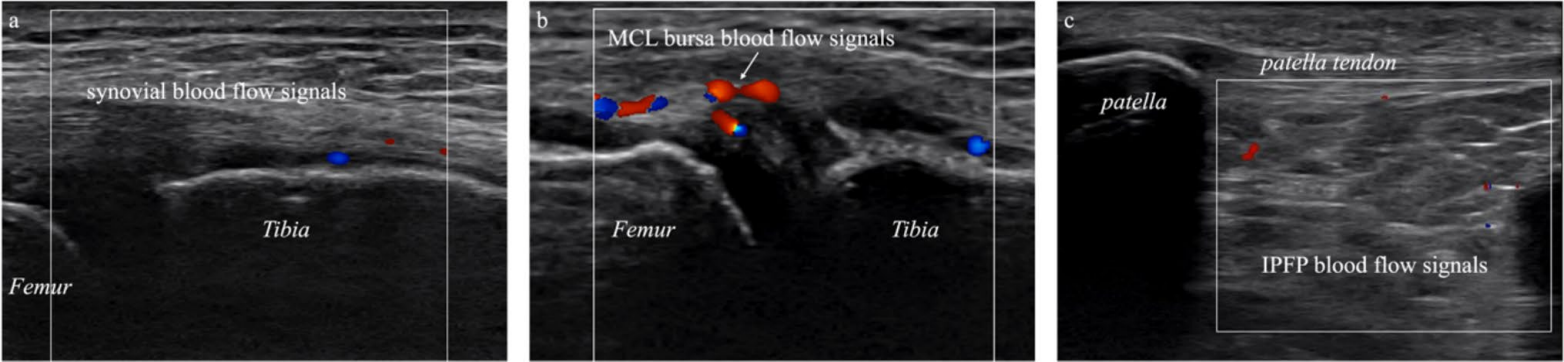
Ultrasonographic findings of synovial, medial collateral ligament bursa, and infrapatellar fat pad blood flow signals The degree of increased blood flow was evaluated in the synovium of the suprapatellar bursa (a), medial collateral ligament bursa (b), and infrapatellar fat pad (c). In accordance with previous studies, the degree of the blood flow signal was classified into four levels (absent, mild, moderate, and marked or severe)
